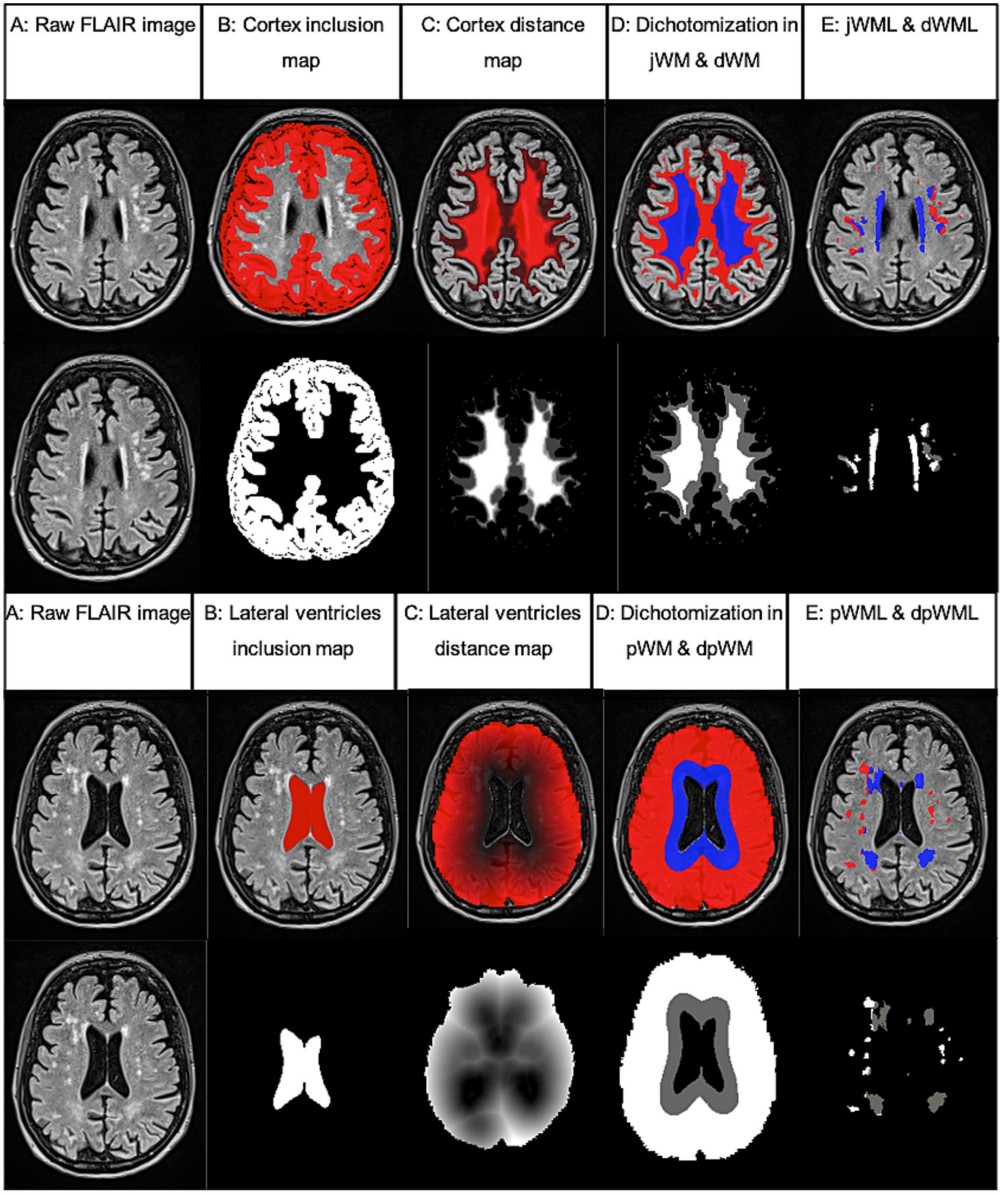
Example of WML segmentation and dichotomization processing steps performed using FSL analysis tools. Based on FLAIR images (A) partial inclusion maps (B) and referential distance maps (C) were calculated and white matter (WM) was dichotomized according to a defined threshold (3 mm from cortex or 10 mm from lateral ventricles, D). Last, the volume of the segmented WML within the dichotomized WM was calculated [juxtacortical (jWML, red) and distacortical (dWML, blue) or deep lobar (dpWML, red) and periventricular (pWML, blue) E]. Inclusion and distance maps (lower rows) are shown as an overlay on the equivalent FLAIR image in the upper rows.
